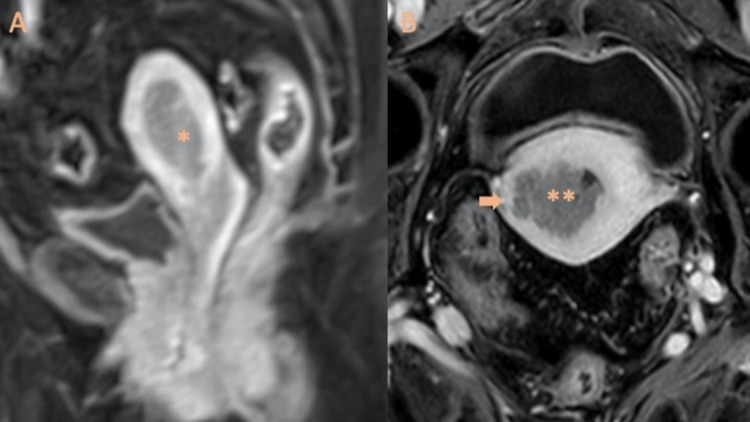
Stage I endometrial cancer A: Stage IA grade 2 endometrioid adenocarcinoma in a 77-year-old woman. Sagittal T1-weighted MRI with intravenous gadolinium-based contrast material demonstrates endometrial tumor (*) confined to the uterine cavity. B: Stage IB grade 3 endometrioid adenocarcinoma in an 82-year-old woman. The extent of myometrial invasion is well delineated after administration of intravenous contrast material. The endometrial carcinoma () infiltrates the outer one-half of the myometrium (arrow).
